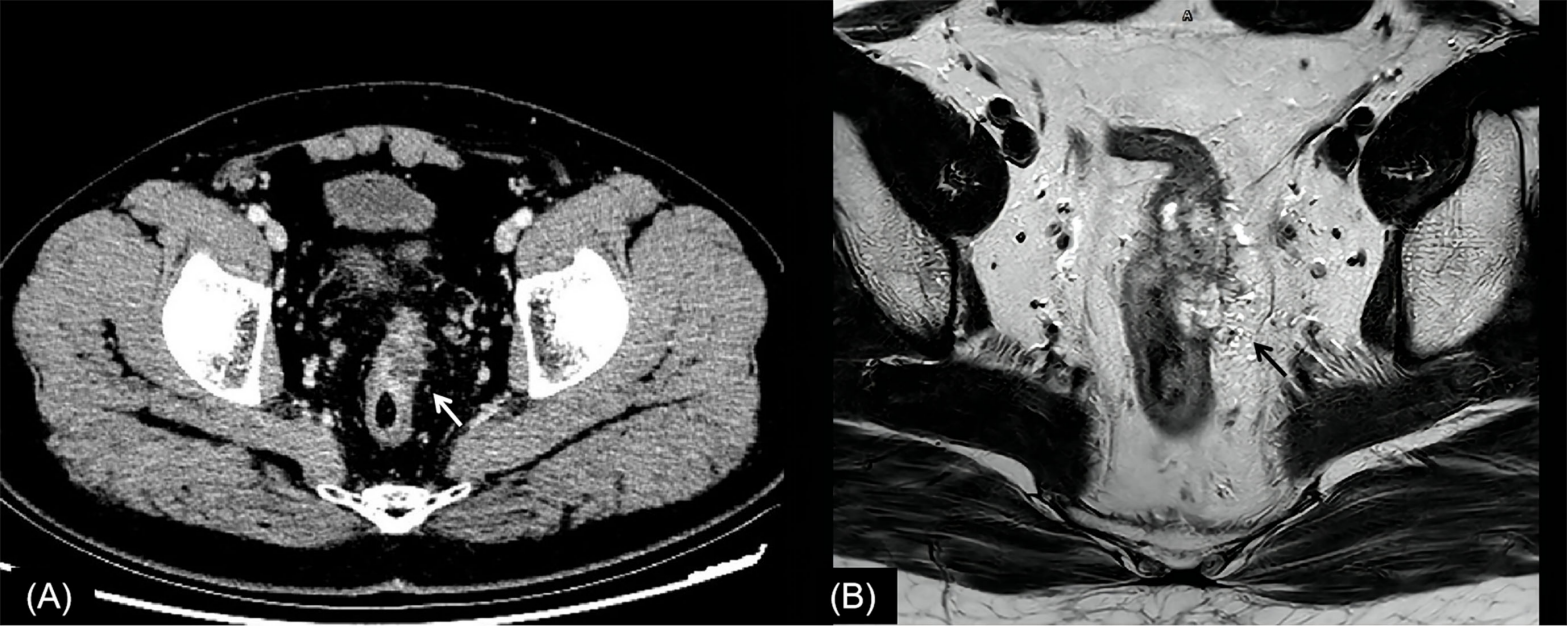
Rectal cancer with ypT0N0. (A) Axial MSCT depicts a heterogeneous enhancing tumor penetrating the peritoneal reflection (arrow). Over restaged as T4. (B) MRI transverse high-resolution T2WI of rectum shows left mesorectal fascia involvement (arrow). Over restaged as T3N0. yp, pathological staging after neoadjuvant chemoradiotherapy; T, tumor; N, node; MSCT, multi-slice computed tomography; MRI, magnetic resonance imaging; T2WI, T2-weighted imaging.
